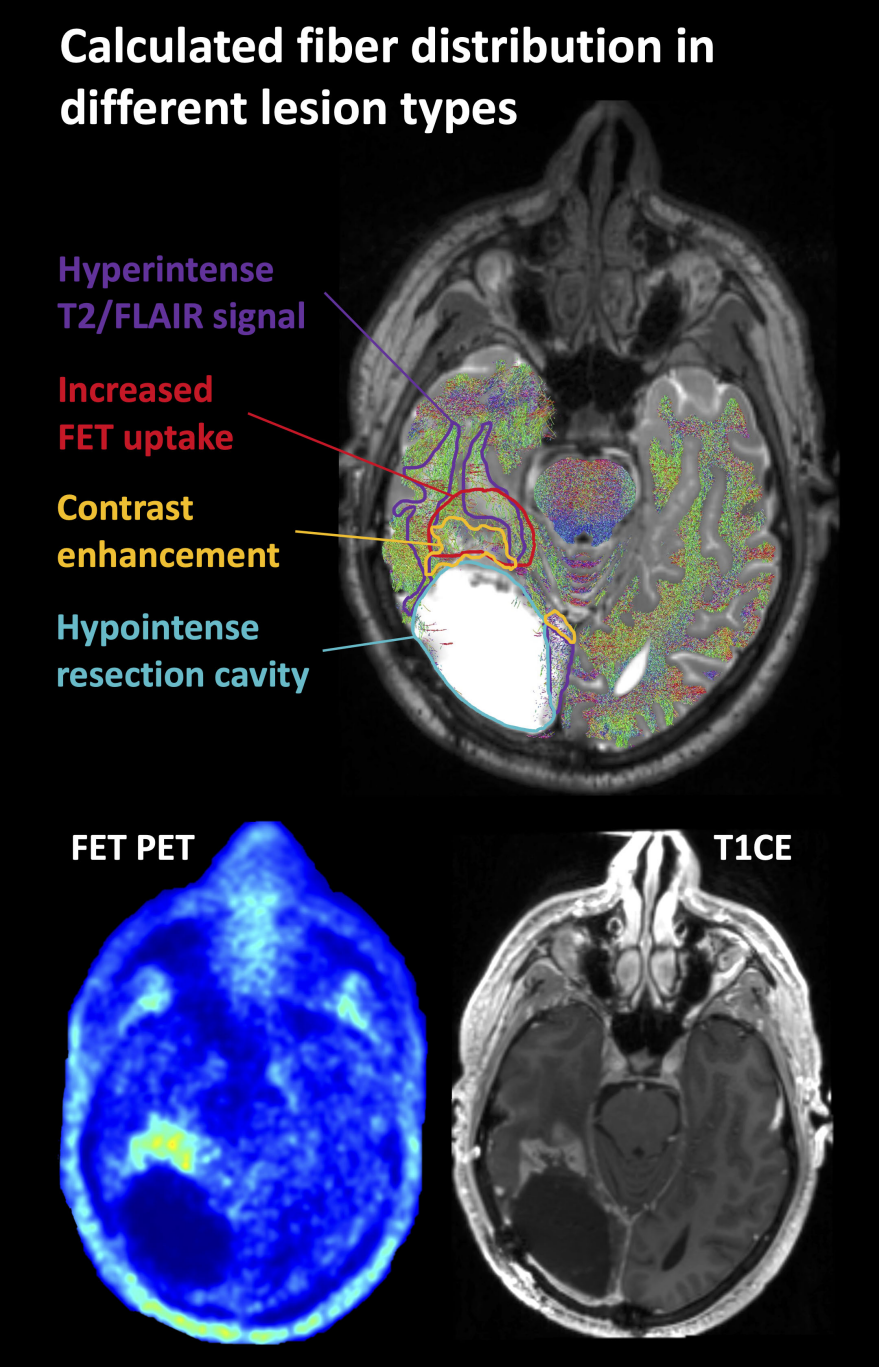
Representative case of lesion segmentation superimposed on a tractography image in a patient who had undergone surgery and radiochemotherapy and developed local recurrence near the resection cavity. FET, O-(2-[^18^F]fluoroethyl)-L-tyrosine; T2/FLAIR, T2-weighted fluid-attenuated inversion recovery; T1CE, T1-weighted contrast-enhancing image.
